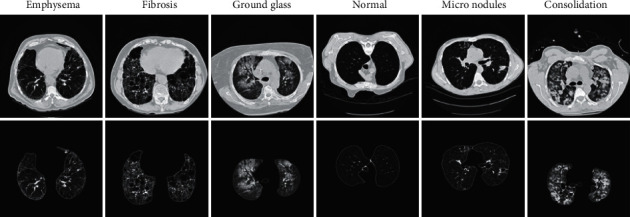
Examples of HRCT of six ILD. (The first row shows original HRCT images and the second row shows respective segmented image.)
